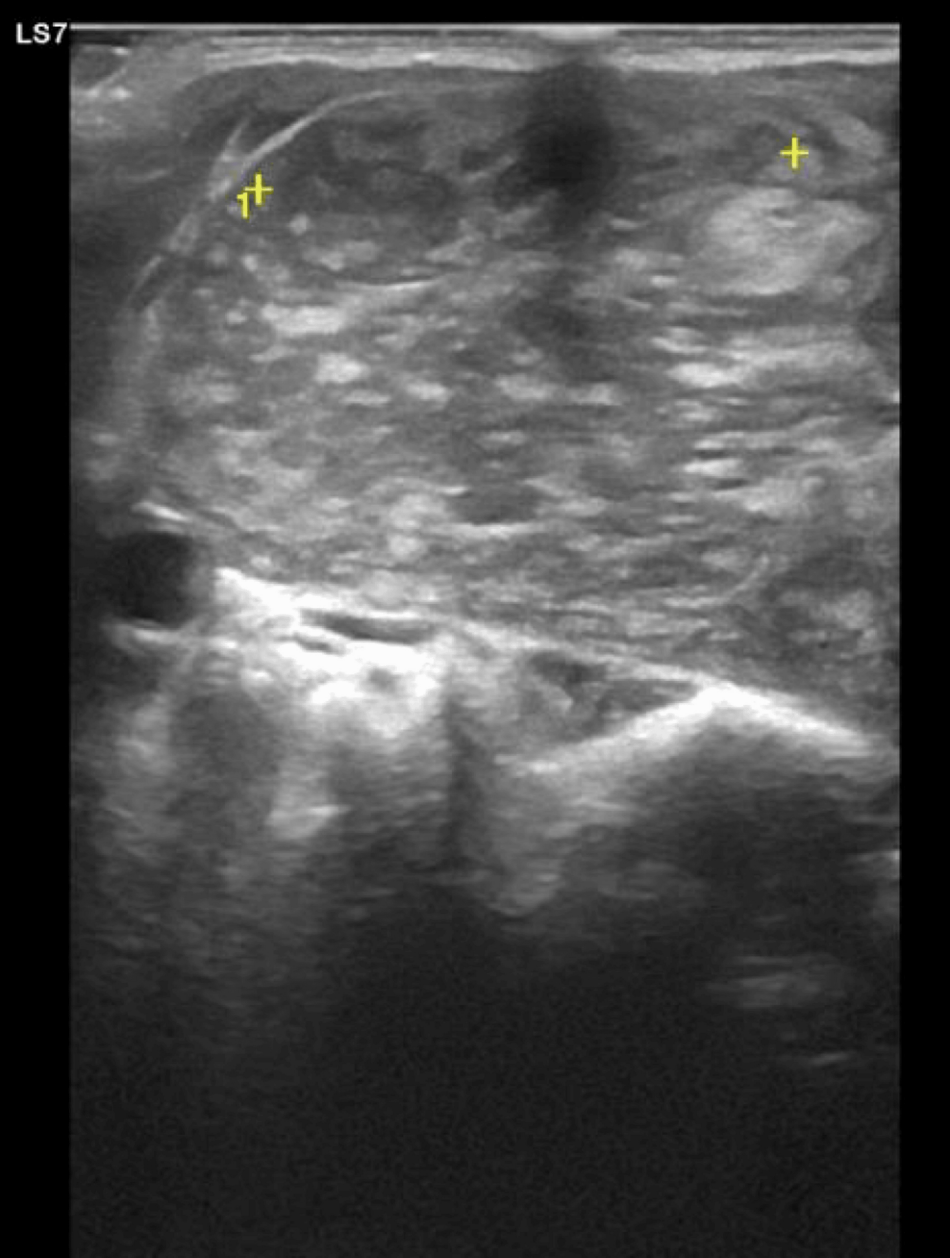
Thyroid ultrasound showing diffuse parenchymal heterogeneity and a vaguely nodular iso/hypoechoic area in the anterior portion of the right lobe, measuring approximately 34 mm.
